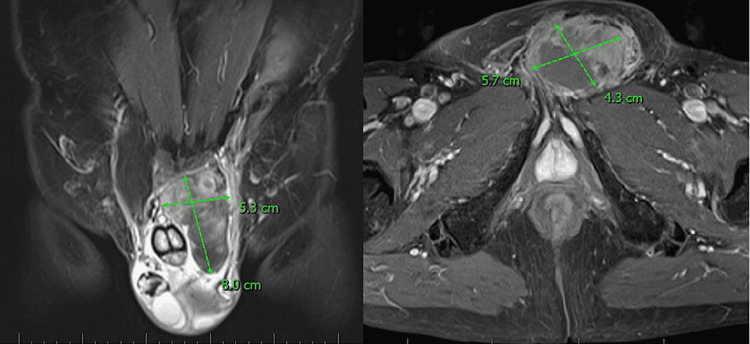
MRI finding of the initial lesion
